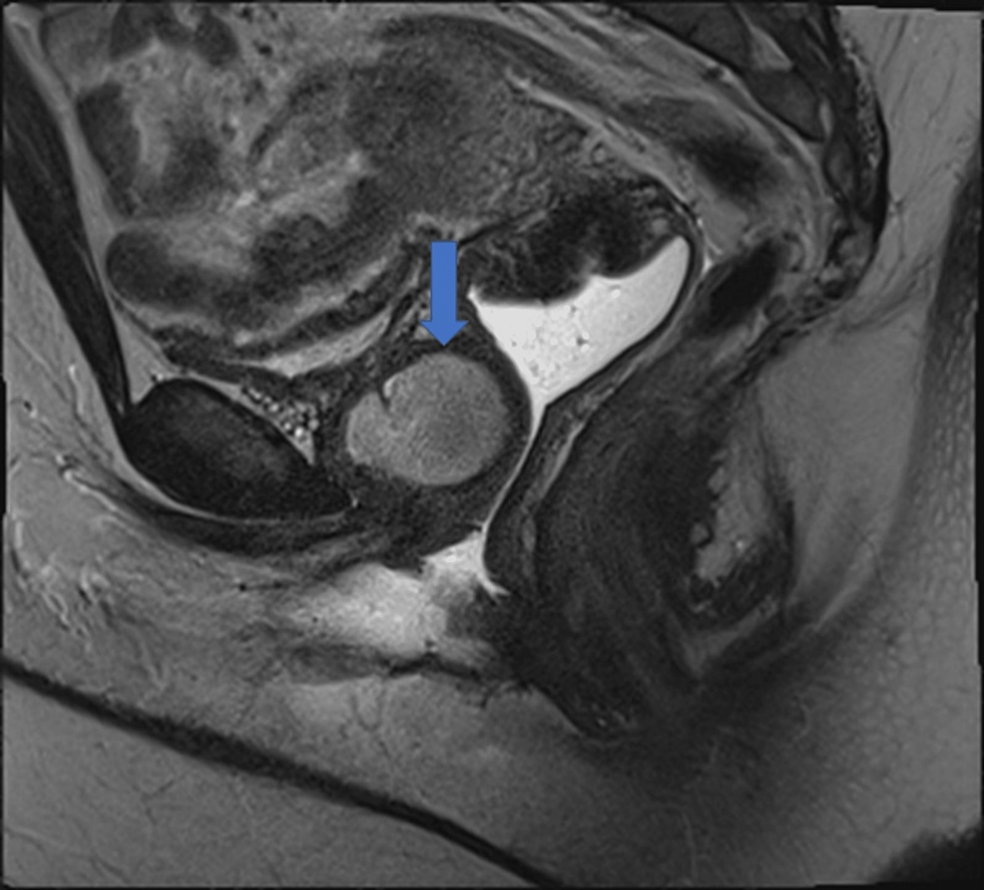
T1 MRI section The image shows a lobulated 3.6 x 2.6 x 3.0 cm lesion (marked with an arrow), likely representing the urethral diverticulum located anterior to the mid-anterior vaginal wall at the level of the pubic symphysis.
